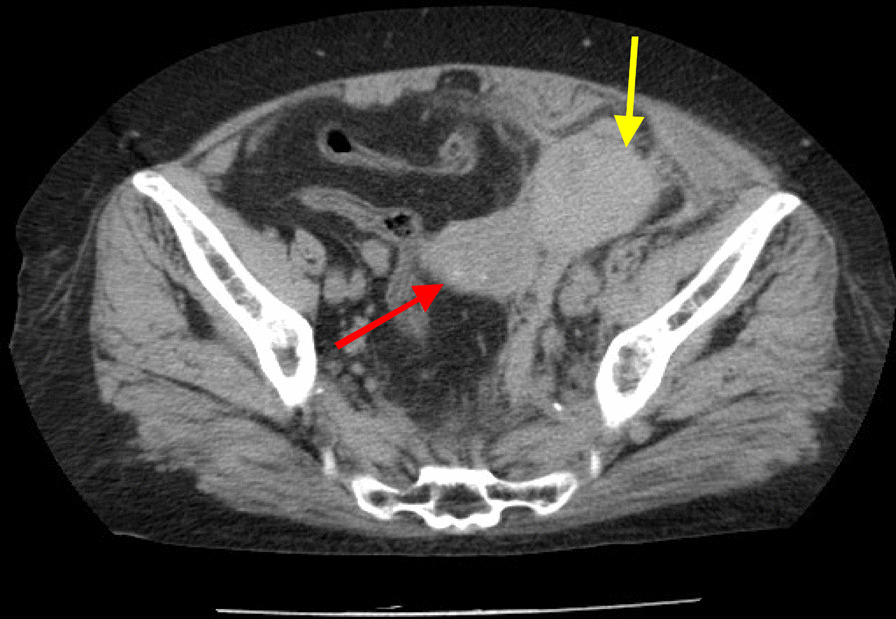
Computed tomographic image at the time of the first hospitalization. The red arrow indicates the uterus and the yellow arrow indicates the left pelvic retroperitoneal hematoma. Initially, this hematoma was diagnosed as a left ovarian cyst
